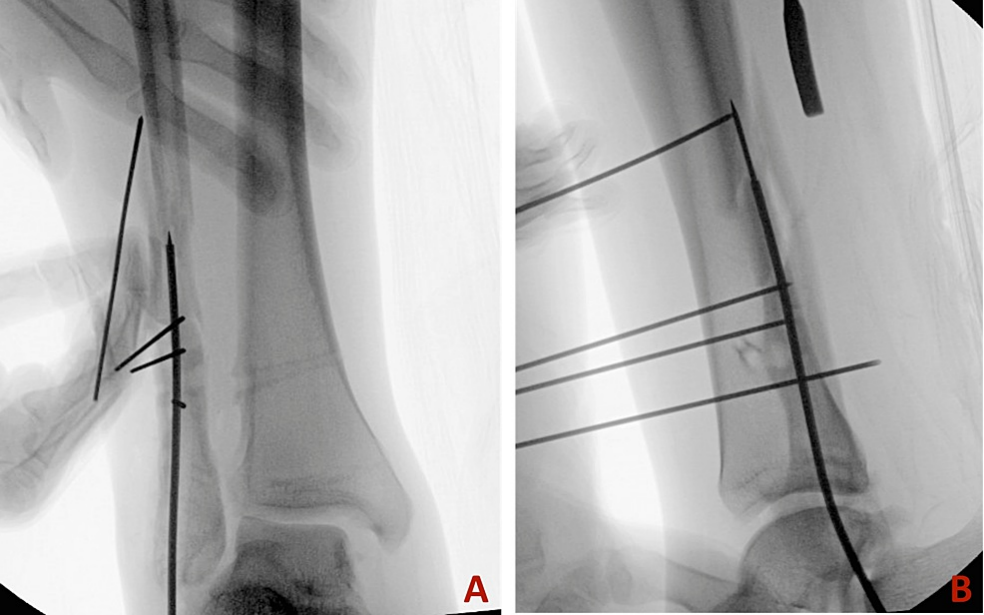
Intraoperative radiographs depicting the use of blocking wires to prevent medial deviation. A: Anterior-posterior view. B: Lateral view
